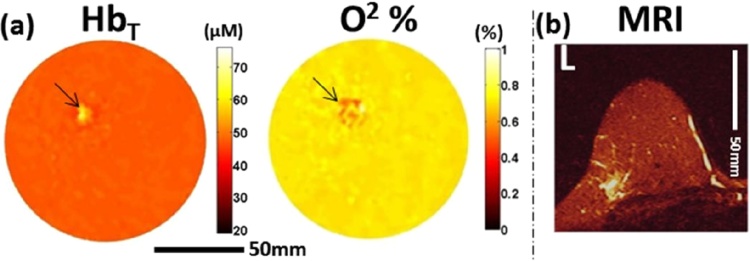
From the University of Florida ring-shaped imager: High grade IDC in the left breast. (A) Coronal HbT and SO_2_ maps from PA imaging through the lesion. (B) 2-D slice of T2-weighted MRI through same region taken 3 days before PA examination. (Reproduced with permission of the authors and publisher.)
